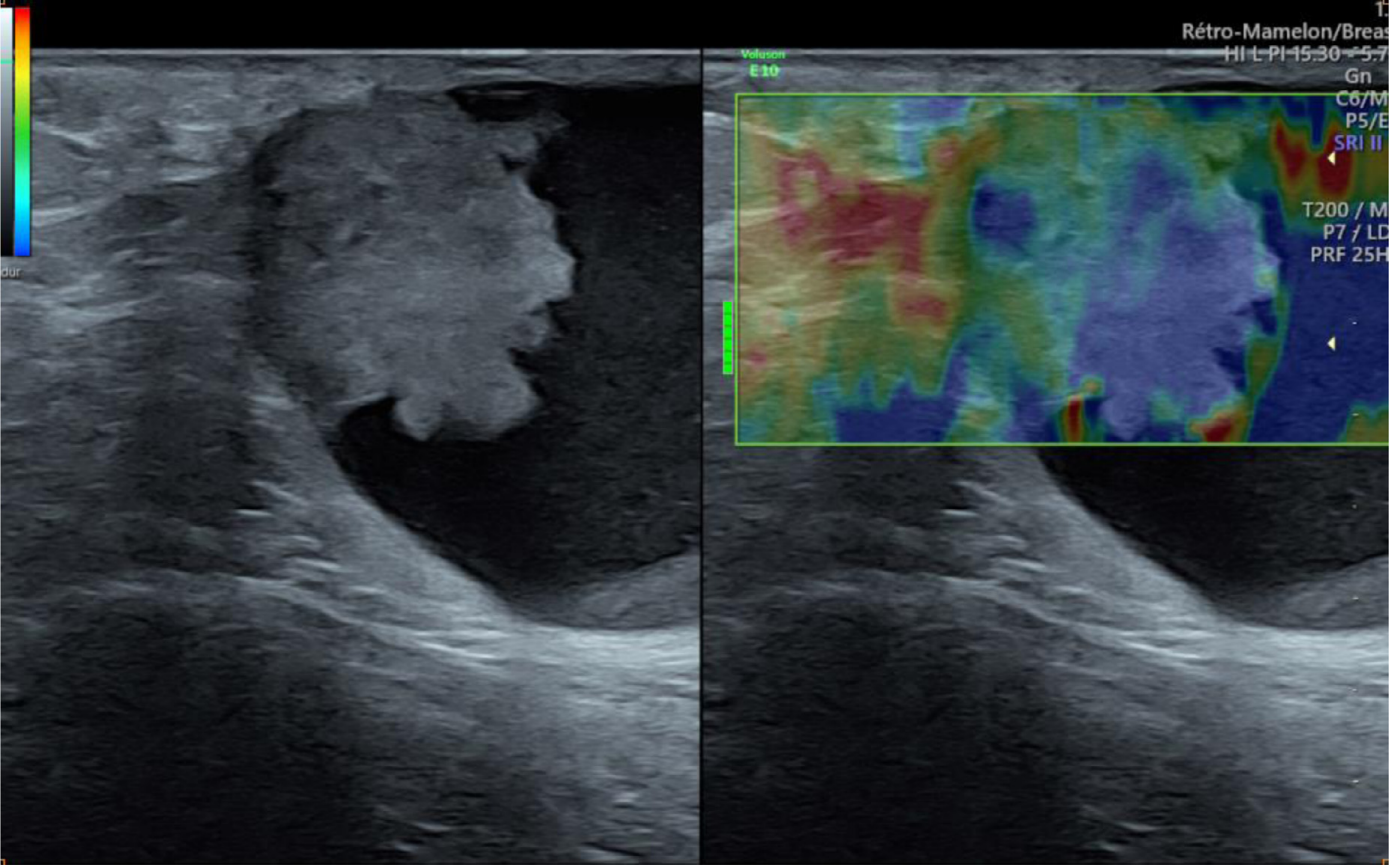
Ultrasound elastography showing hard elasticity of the tissue component.
